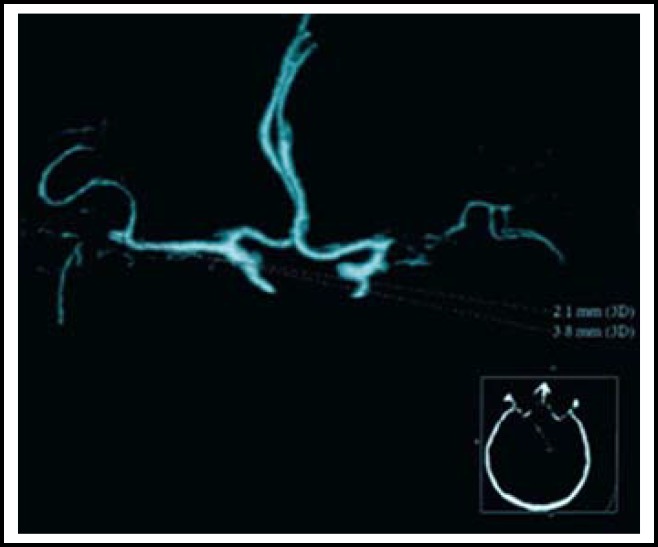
Admission CTA of the patient shows right MCA M1 occlusion and left MCA bifurcation stump, the stump measured 2.1 mm × 3.8 mm on CTA volume-rendered image
